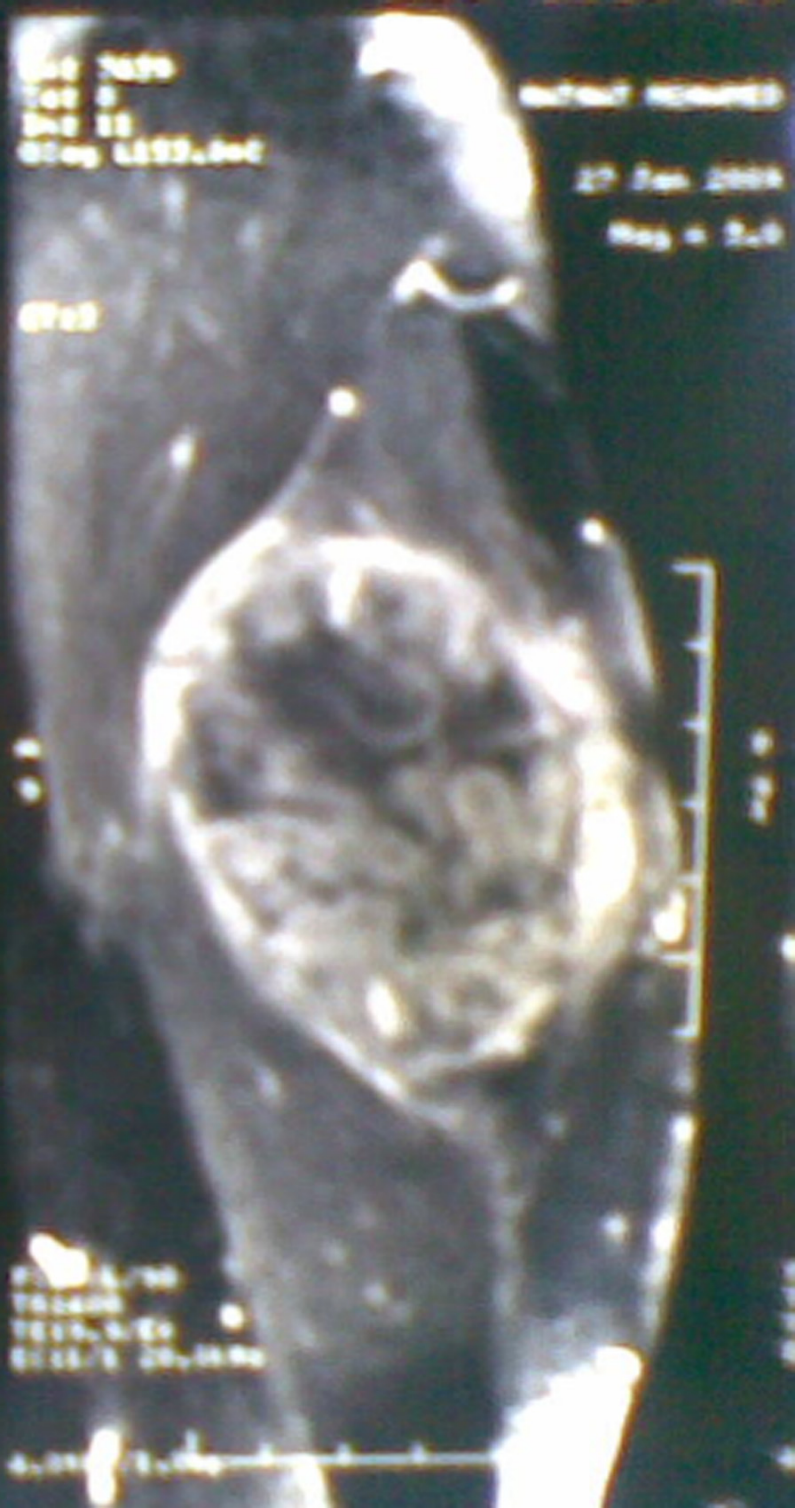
MRI image after administration of gadolinium-diethylenetriaminepentacetate (Gd-DTPA) (coronal) showing a 53 × 40 mm diameter soft tissue mass in the posterior compartment of the right arm.
